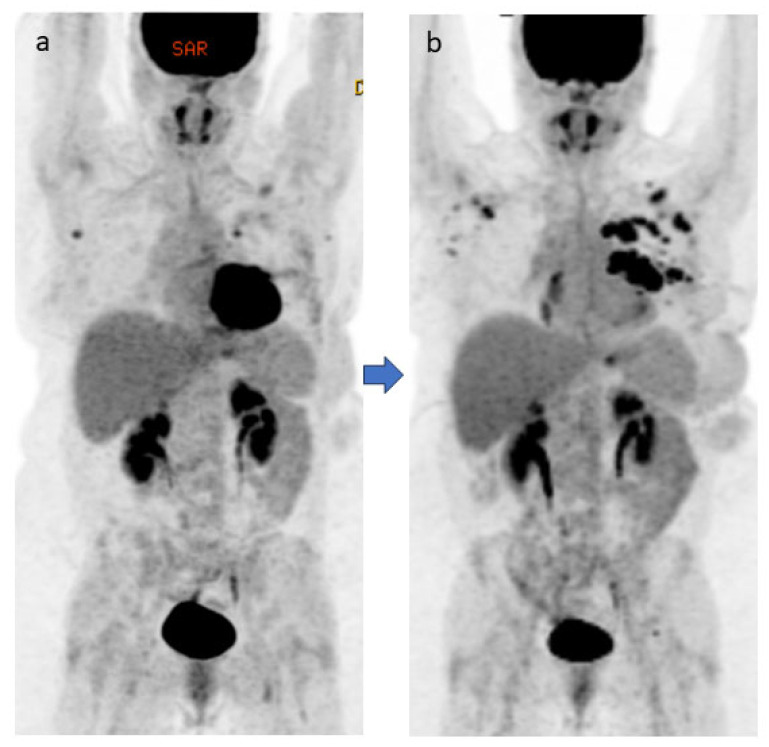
A female patient with metastatic left breast carcinoma and receiving immunotherapy. Neither the PET/CT completed 1 month before the colitis episode (maximum intensity projection [MIP] image (a)) nor the one completed 1 month after the colitis episode (MIP image (b)) showed any appreciable abnormal increased uptake in the colon.
